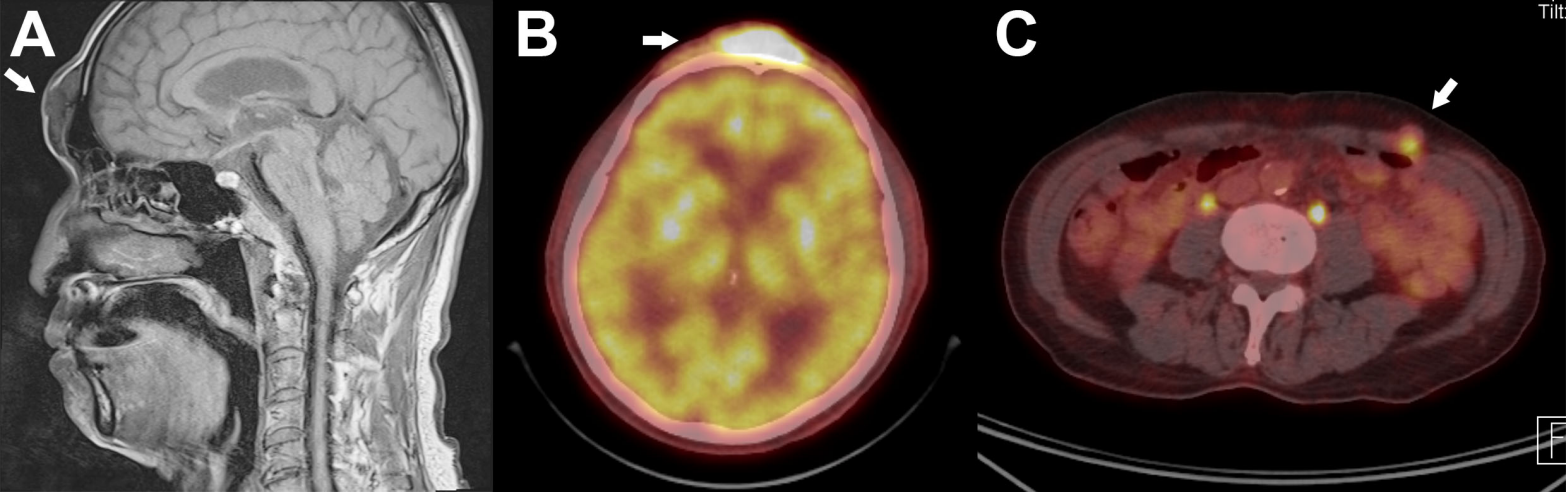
Magnetic Resonance Imaging(MRI) and positron Emission tomography(PET) Scan. (A) T1-Weighted sagittal view shows nodular accumulation of foreign body material in the subcutaneous layer of the forehead. (B) PET-CT scan shows high metabolic activity (SUVmax=15) in the same region as shown in (A). (C) Hypermetabolic activity was also noted in the anterior abdominal wall, suggesting distant migration of the injected material. Biopsy confirmed the same pathology with the lesion in (A).
